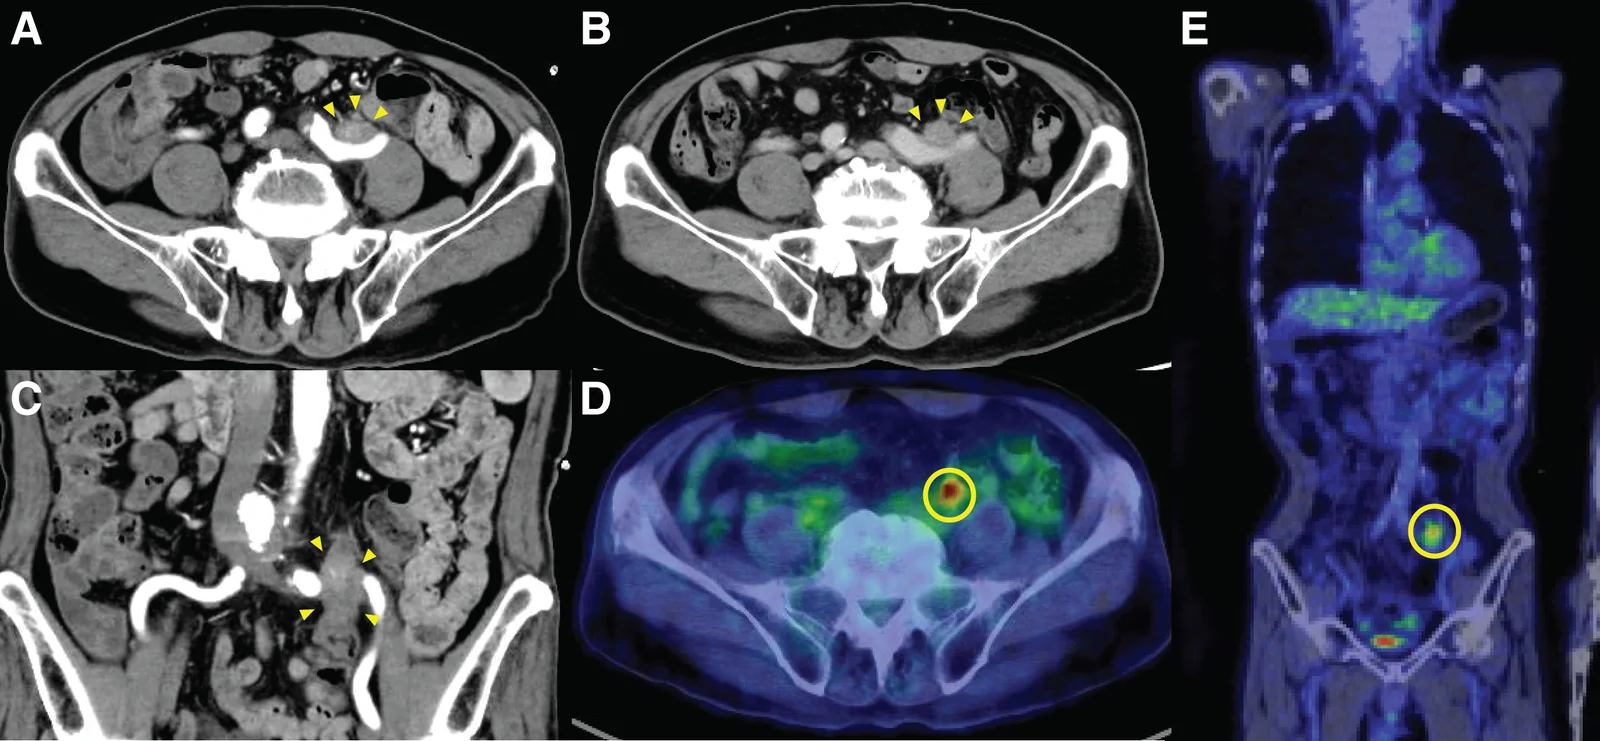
(A, C) Preoperative contrast-enhanced CT showing interval enlargement to 25 mm without overt iliac vessel invasion. (B) Contrast-enhanced CT at the time of initial recurrence detection showing a 20-mm pelvic mass anterior to the left iliac bifurcation. (D, E) Axial and coronal PET/CT images, respectively, demonstrating focal FDG uptake in the pelvic lesion and no distant metastasis. FDG, fluorodeoxyglucose
